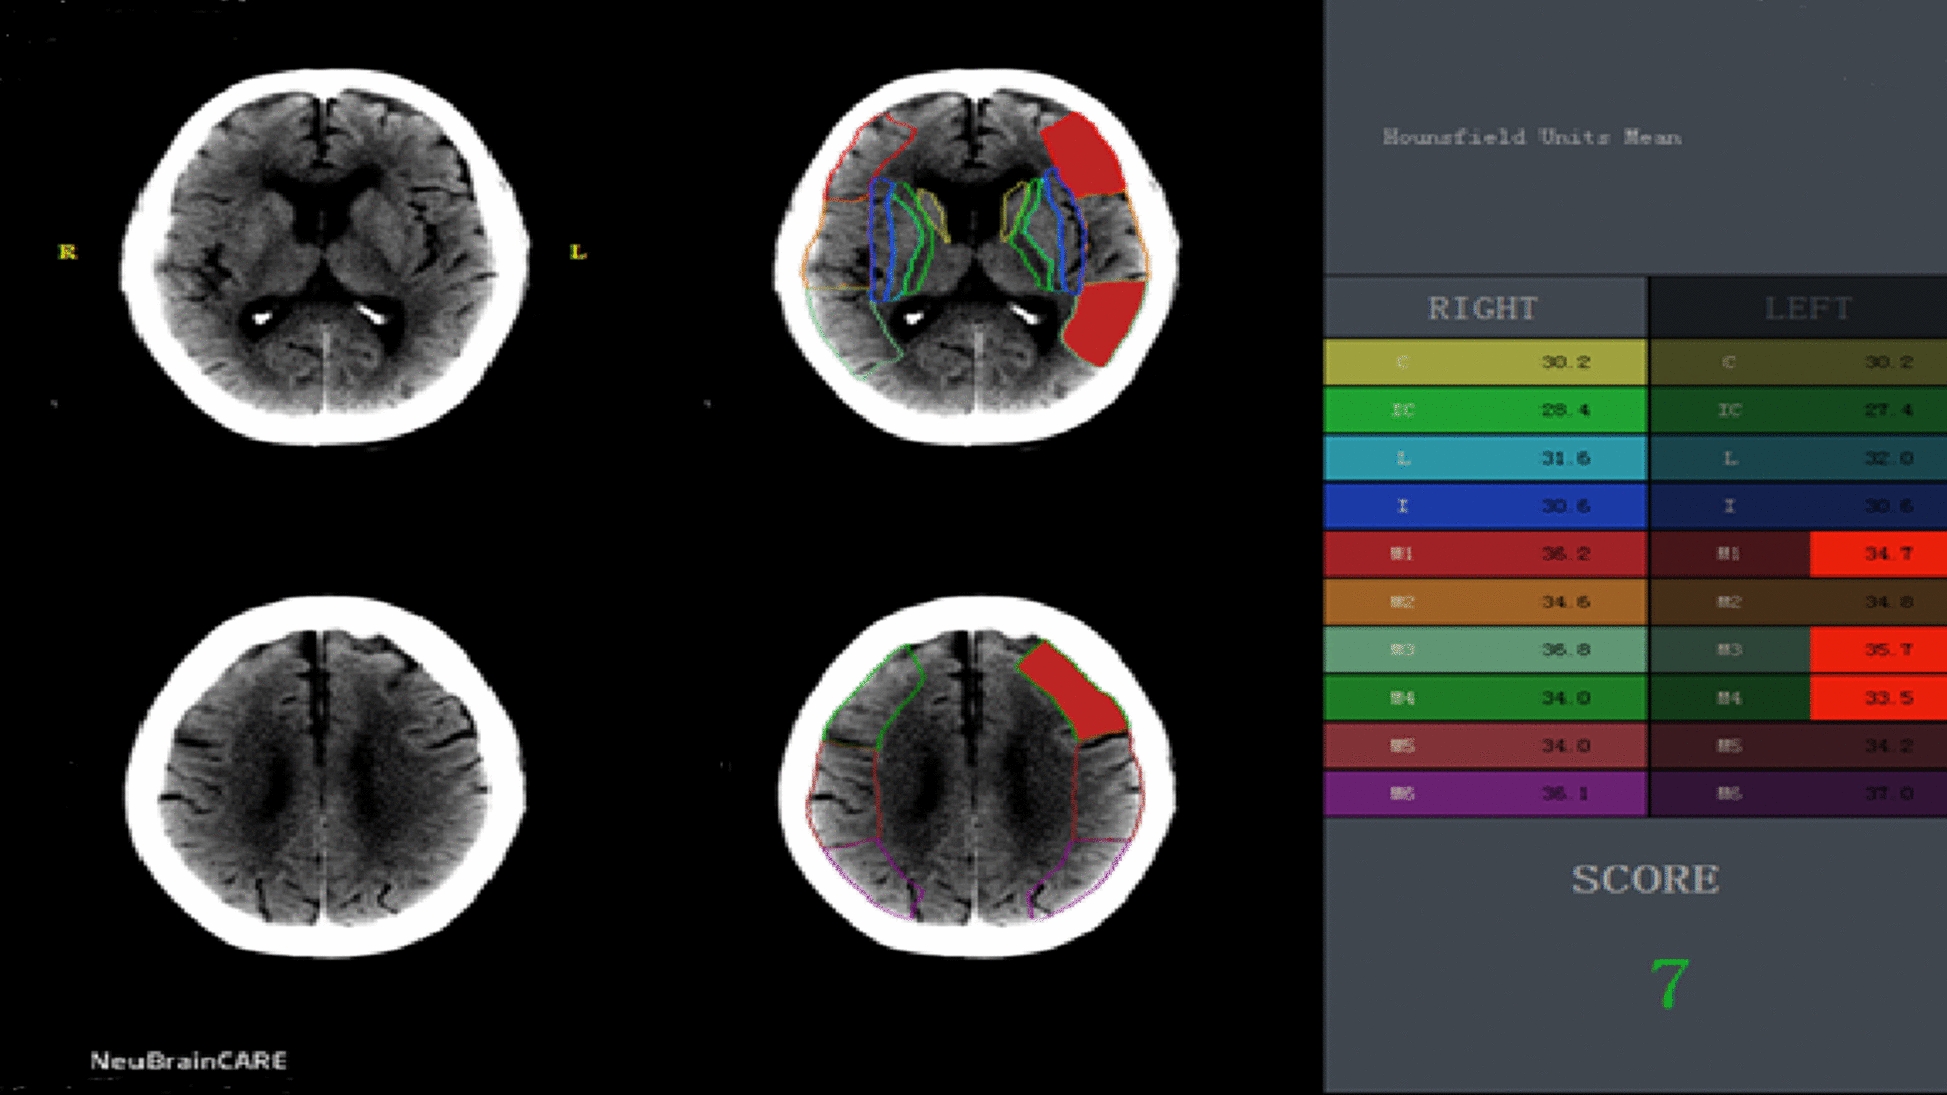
Automatic ASPECTS of CT plain scan images by NeuBrainCARE. The automatic ASPECTS was obtained after the correction and evaluation of CT plain scan images by analysis using NeuBrainCARE, which marks the red area as the affected area, with the automatic ASPECTS value (score 7)
